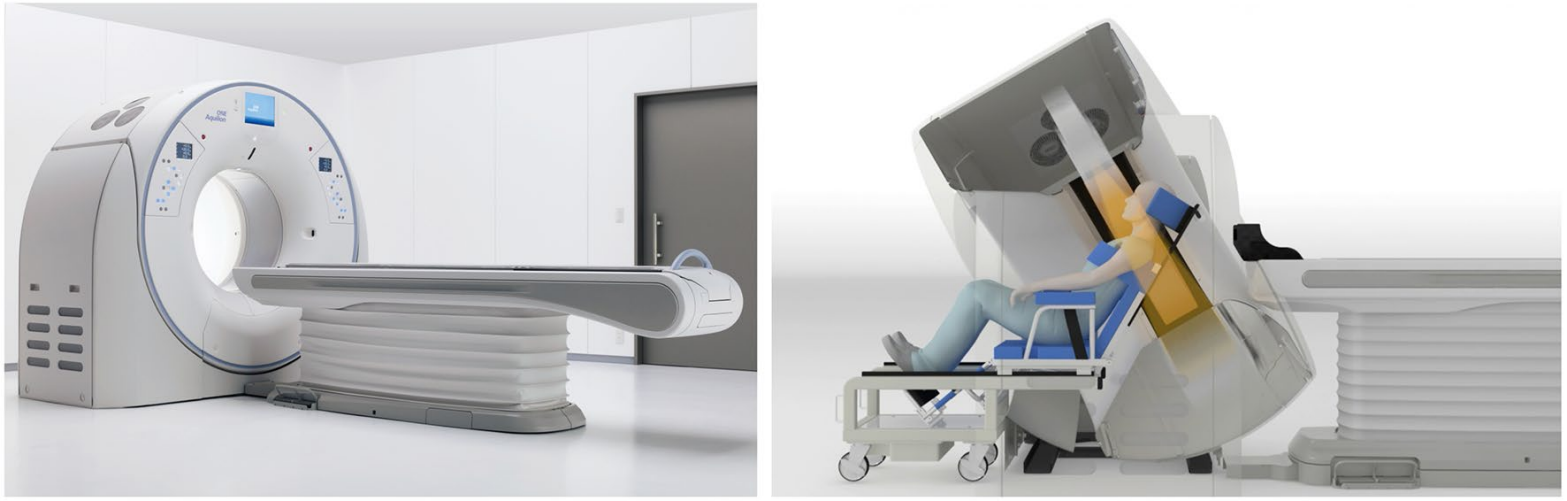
Aquilion ONE (Canon Medical) and reclining position in 320-row area detector computed tomography (320-ADCT) using offset-Sliding CT Chair. Left: Aquilion ONE Right: reclining position—30° from the upright
